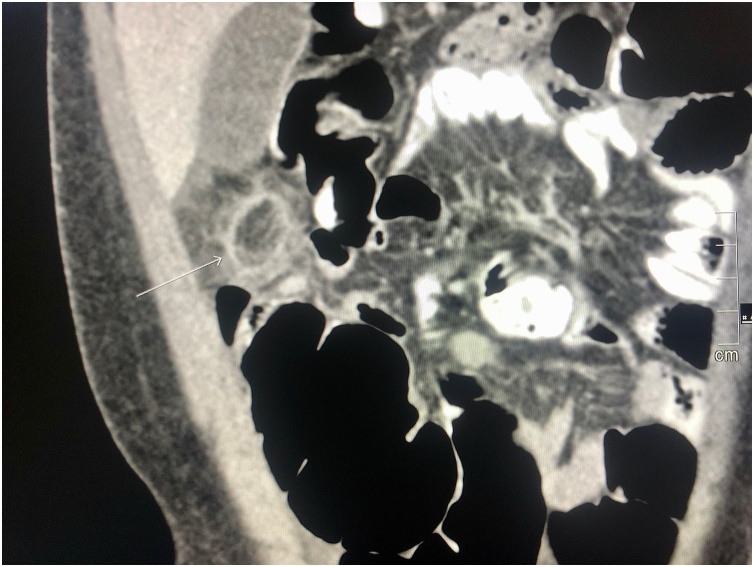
Abdominal CT scan with contrast showing an ovoid fat structure with thin enhancing rim and inflammatory stranding with free fluid in the pelvis.
